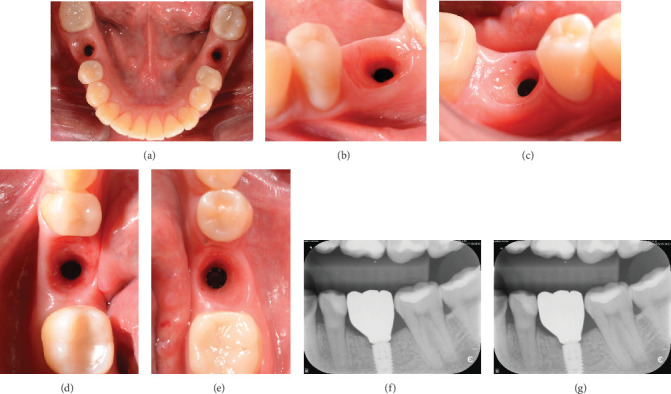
(a) Control 1 month after crown delivery; (b) lateral view, peri-implant mucosa of Implant 19; (c) lateral view, peri-implant mucosa of Implant 30; (d) occlusal view, peri-implant mucosa of Implant 19; (e) occlusal view, peri-implant mucosa of Implant 30; (f) periapical x-ray of Zone 19, 1 month after crown screwing; (g) periapical x-ray of Zone 30, 1 month after crown screwing.
